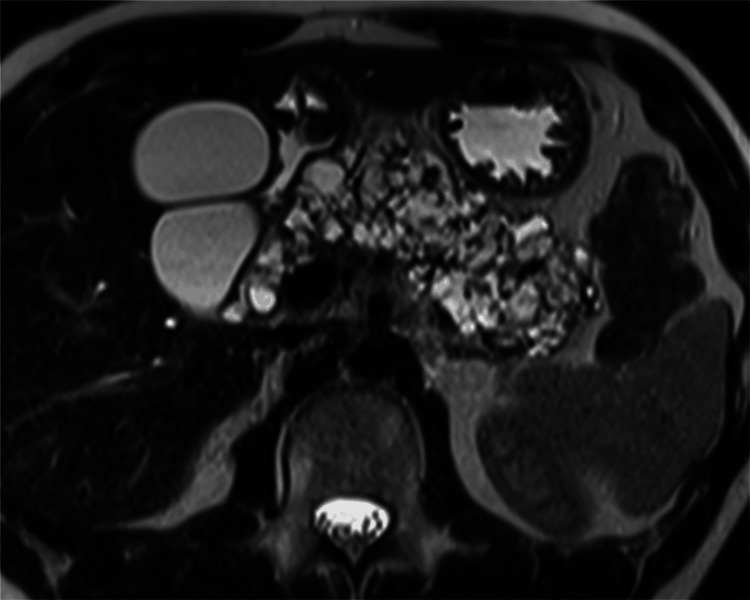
An axial heavily T2-weighted magnetic resonance image of a 36-year-old man with insulin-dependent diabetes mellitus (Patient 4 in Table 3) The percentage of pancreatic cysts is 72.1%.
